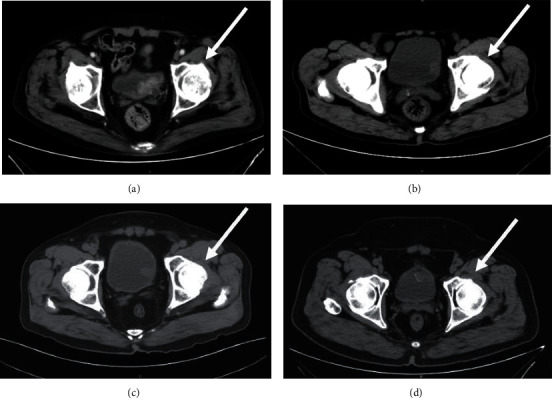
CT medical image of the patient depicting the following: multiple lesions in the bladder (a); round bulge-like lesions in the bladder (b); bladder wall unevenness at the junction of the lesions (c); plaque calcification in the lesions (d).
